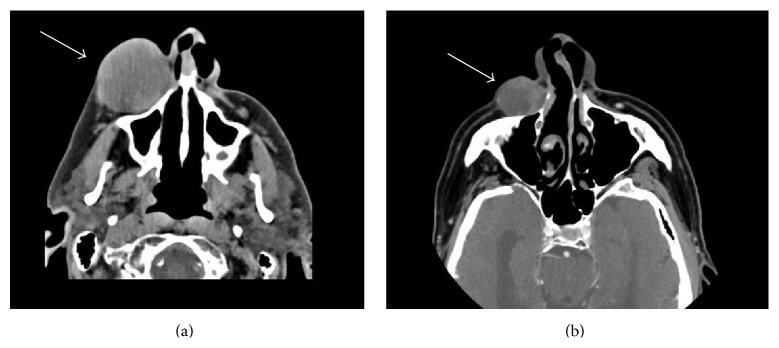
Radiographic images. Radiographic findings of facial bone computed tomography (CT) scan at time of initial presentation (a) and following 4 months of vismodegib therapy (b).
